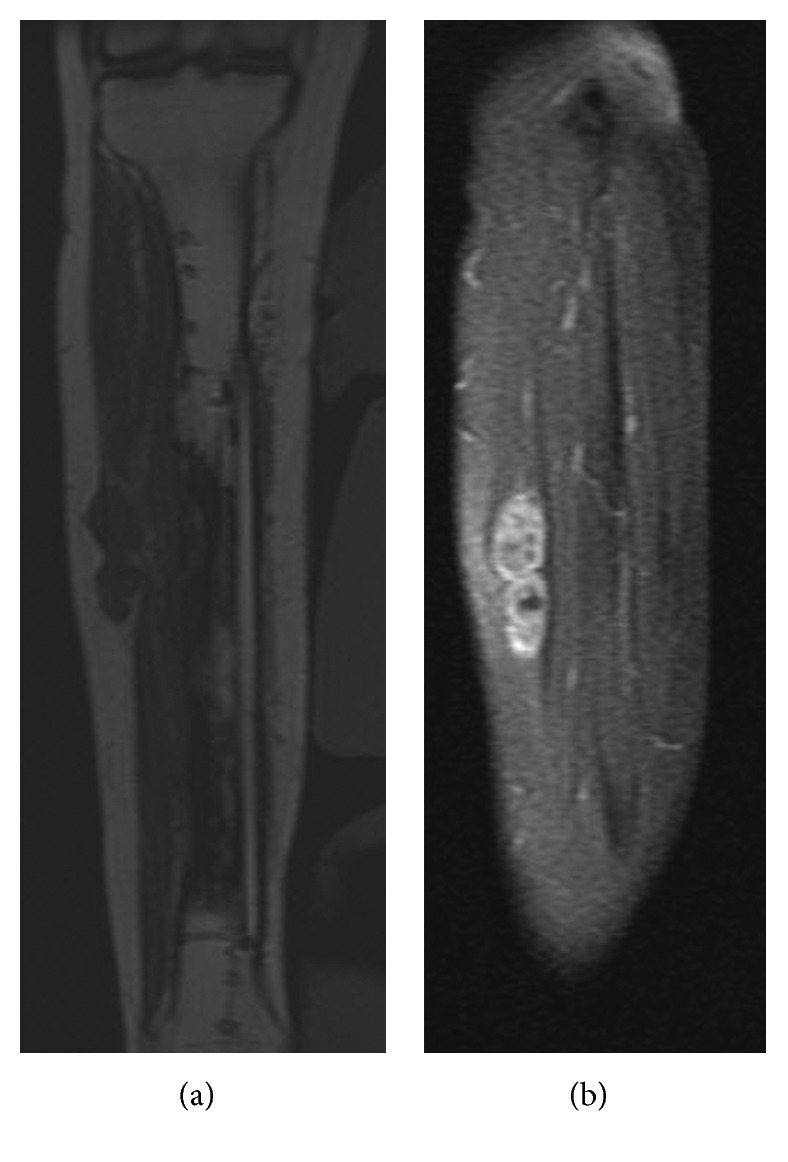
(a) A coronal T1-weighted magnetic resonance image showing a dumbbell-shaped mass in the anterolateral subcutaneous tissues involving the underlying extensor hallucis longus and extensor digitorum longus muscles. (b) The mass enhances diffusely after gadolinium administration, consistent with a soft tissue mass.
